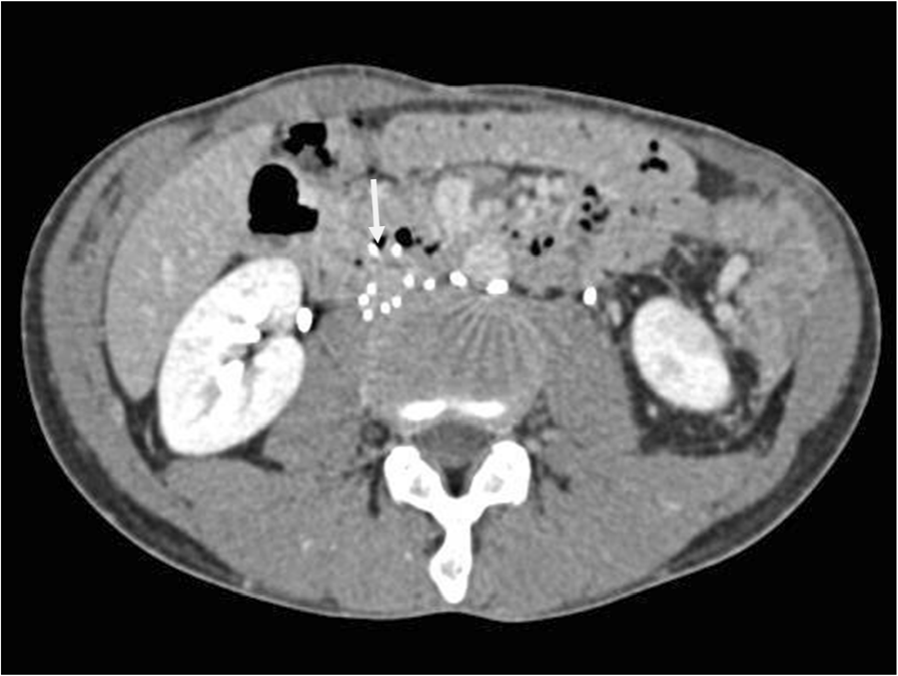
Computed tomography (CT) revealed that IVC filter strut penetrated the third portion of the duodenum (arrow)
